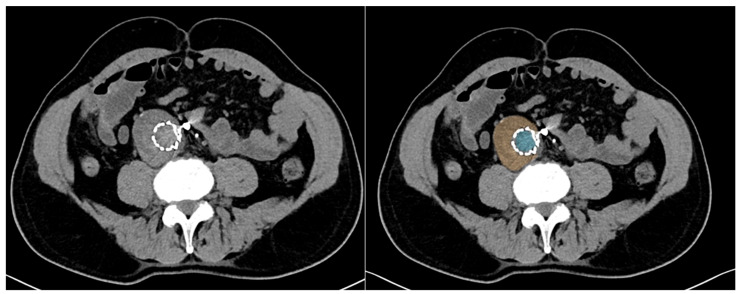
Axial CT images acquired after EVAR without contrast medium. On the left, the same slice is shown after segmentation of the lumen (light blue) and aneurysm sac (orange), defining the regions of interest for radiomic feature extraction and subsequent AI-based analysis.
